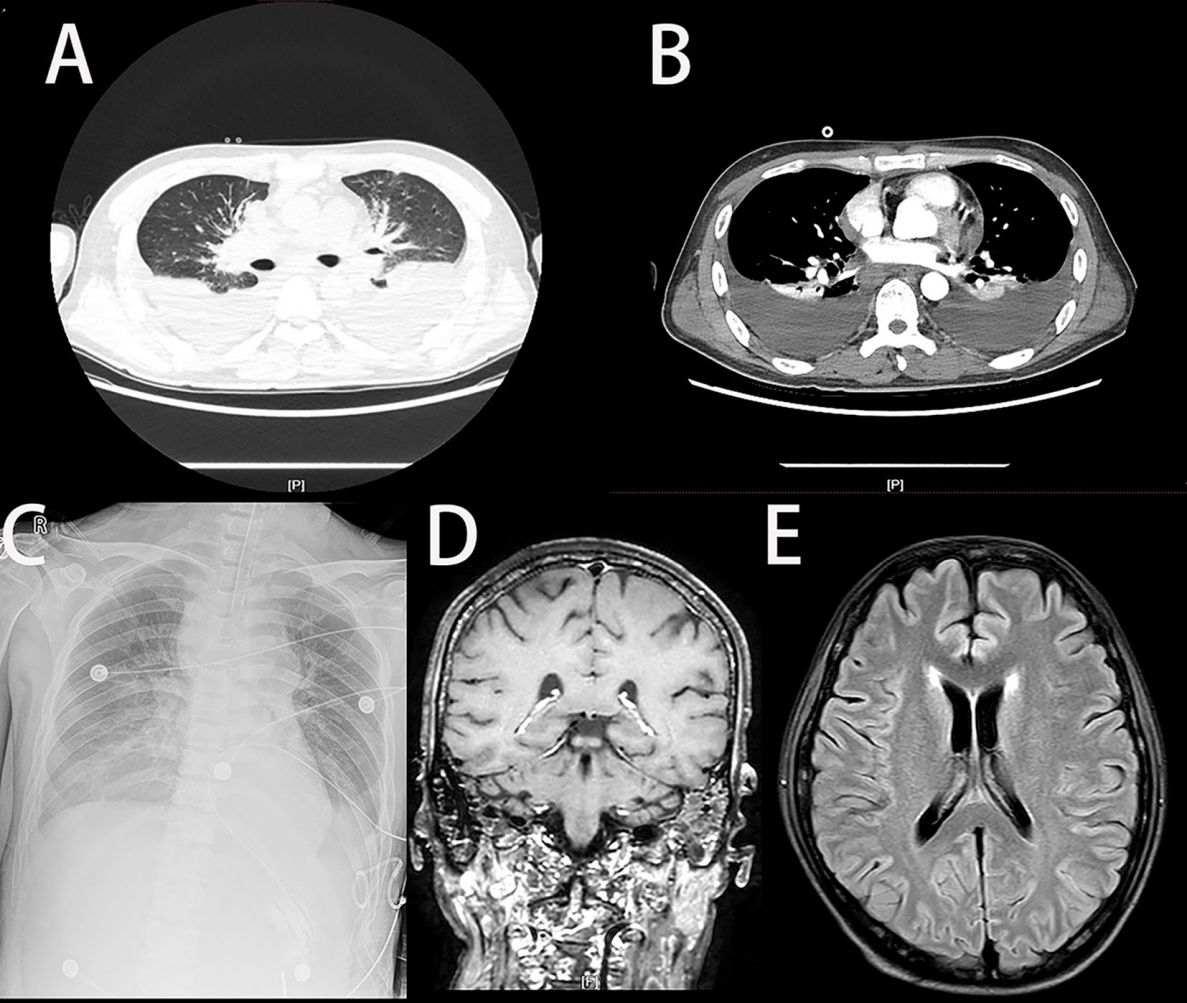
Admission imaging findings. Radiologic studies upon admission showed pleural effusion and pulmonary exudates, consistent with infection. (A) Axial non-contrast chest CT (lung window). (B) Axial non-contrast chest CT (mediastinal window). (C) Posteroanterior (PA) chest radiograph. (D) Coronal brain MRI. (E) Axial brain MRI (FLAIR sequence).
